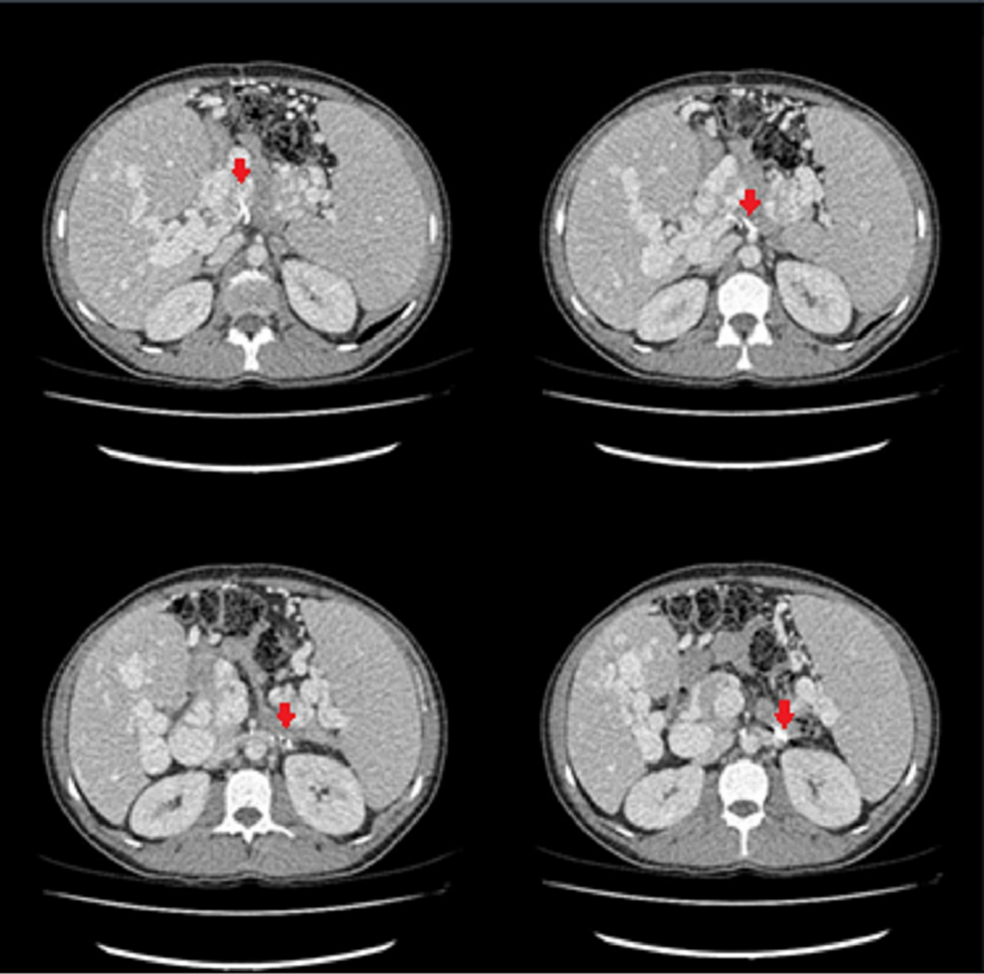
Computed tomography showing mesenteric-inferior-renal shunt placement (red arrows).
